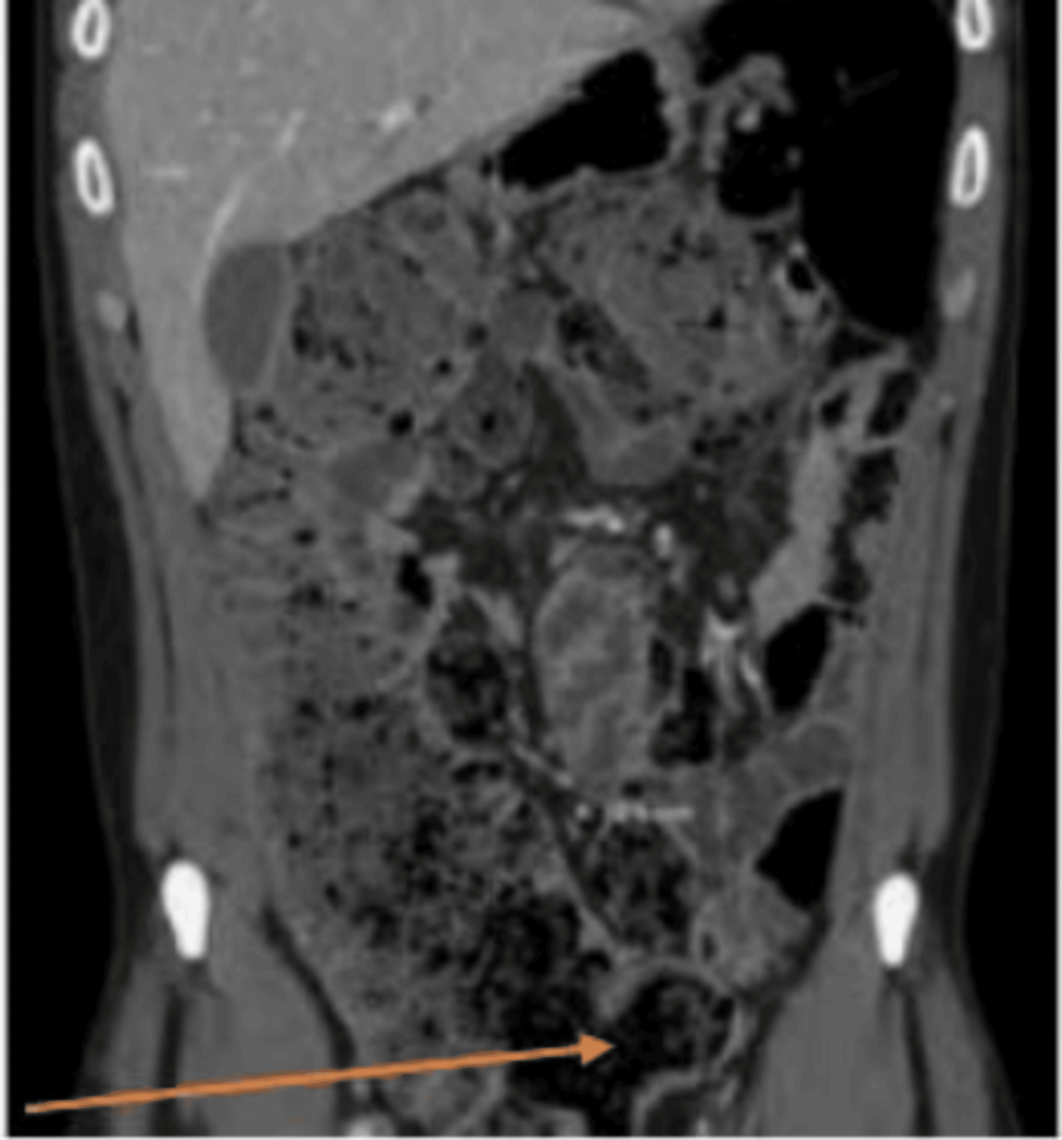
CT of the abdomen before treatment. The arrow shows the fecal retention.
